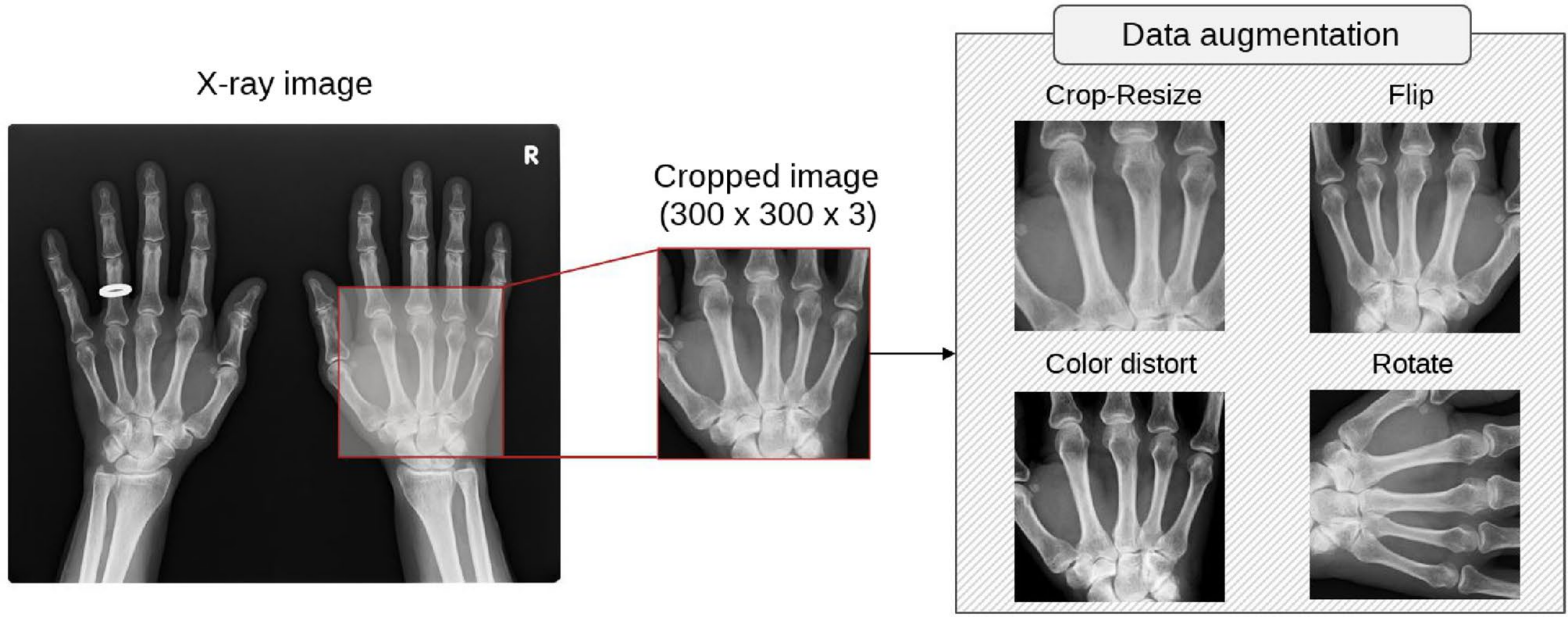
Input image pre-processing during the training phase. The X-ray image is randomly cropped as an input image for the SSD and is augmented by coloring, crop-resizing, rotating, and flipping.
